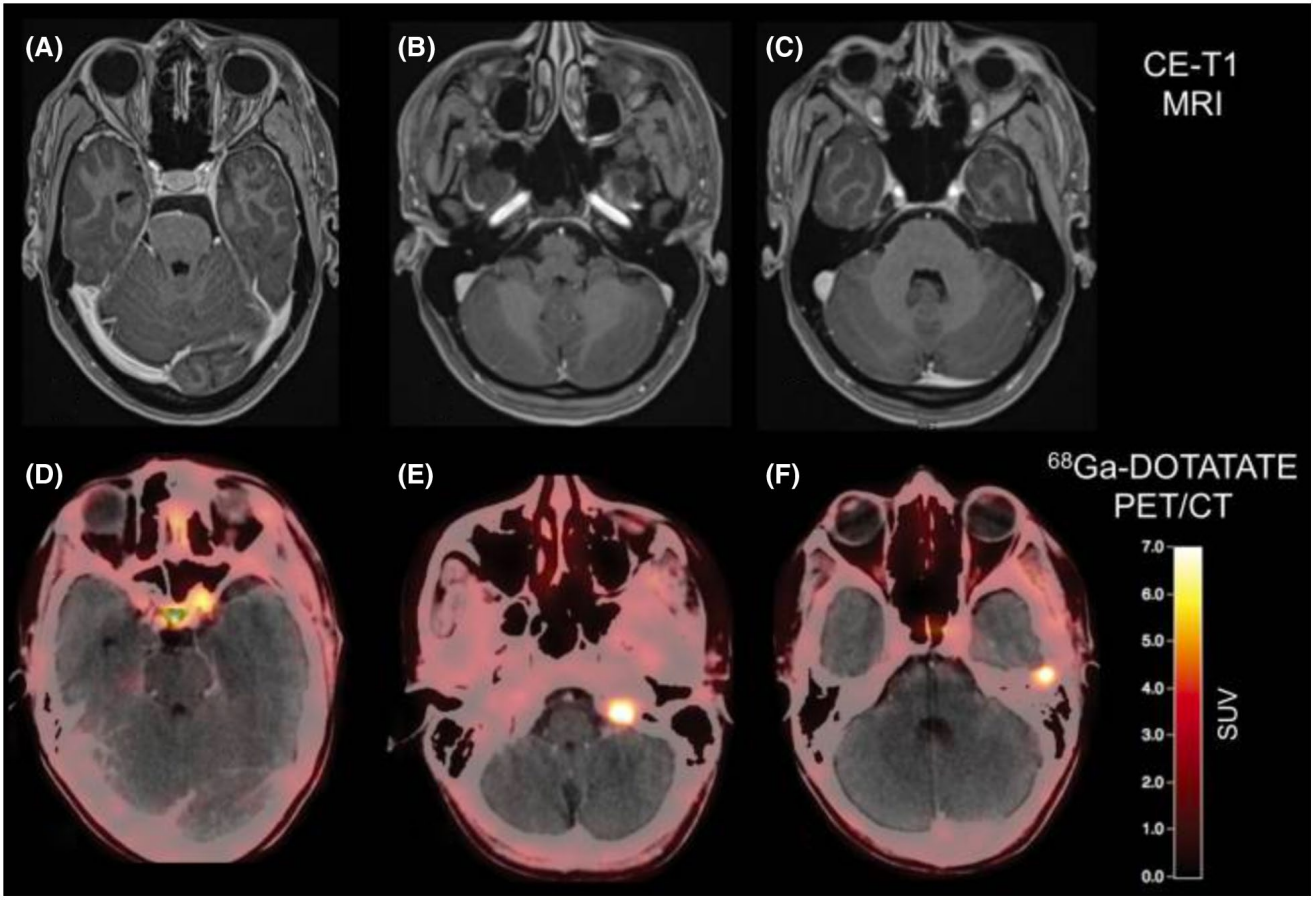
Postoperative contrast‐enhanced MRI and DOTATATE PET/CT of a patient after resection of a WHO grade I meningioma show residual tumor located at the left internal carotid artery and a tumor at the tip of the left orbit (A and D). Surprisingly, two additional meningiomas were also visible on the DOTATATE PET/CT (E and F), without corresponding contrast enhancement on MRI (B and C) (reproduced from Galldiks et al. [33], with permission from Oxford University Press)
